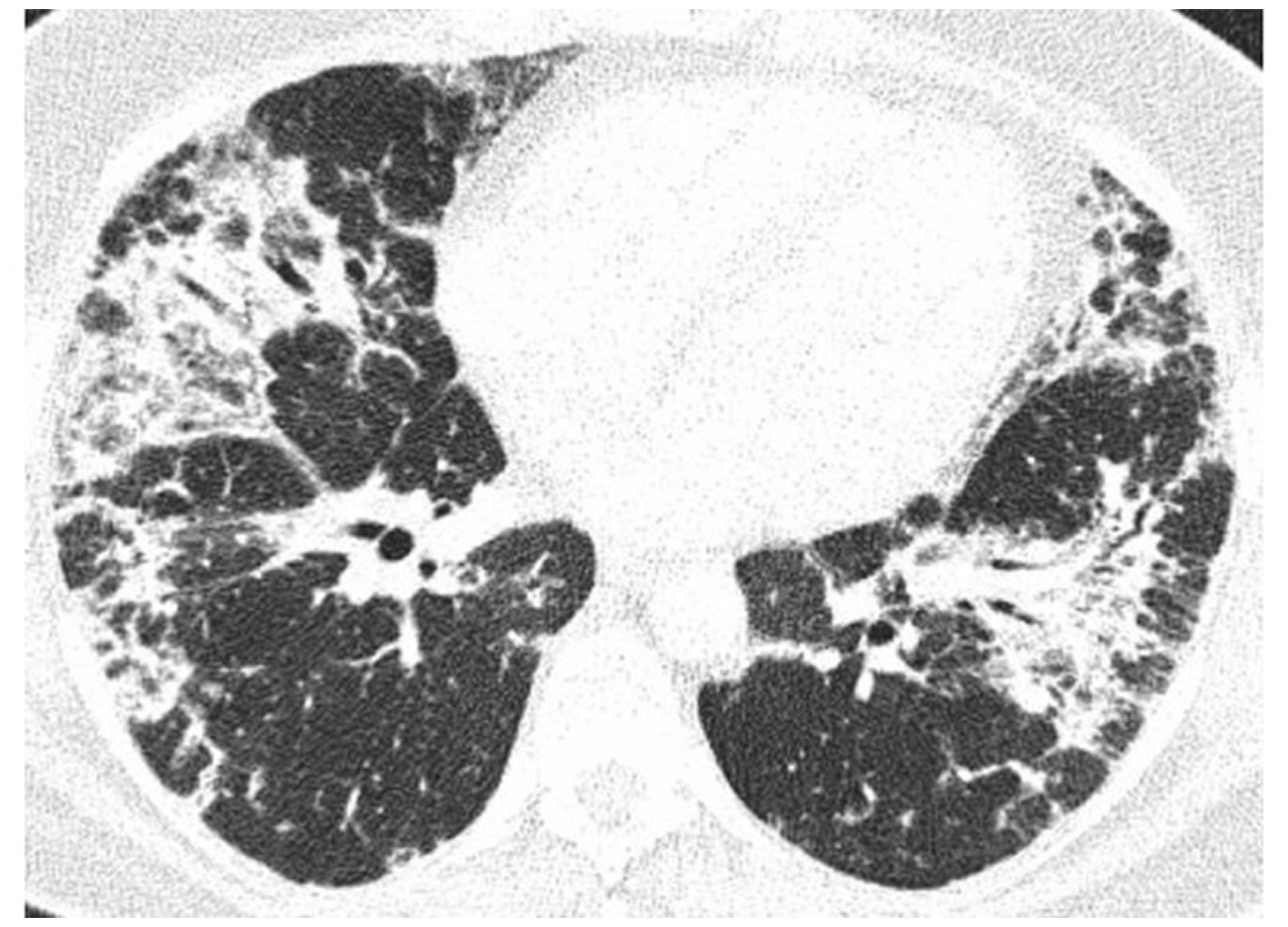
HRCT scan. Etanercept induced AFOP, in patient with rheumatic arthritis and pulmonary fibrosis. Bilateral consolidations with air bronchogram, interlobular septa thickening, and ground glass opacities predominated in the peripheral parts of both lungs.
